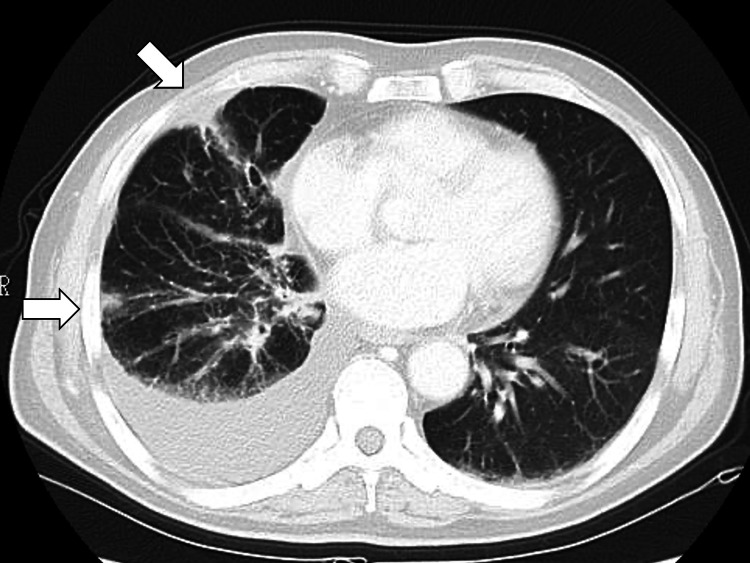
Chest computed tomography showing continued response at the primary site of the lung (white arrows)
